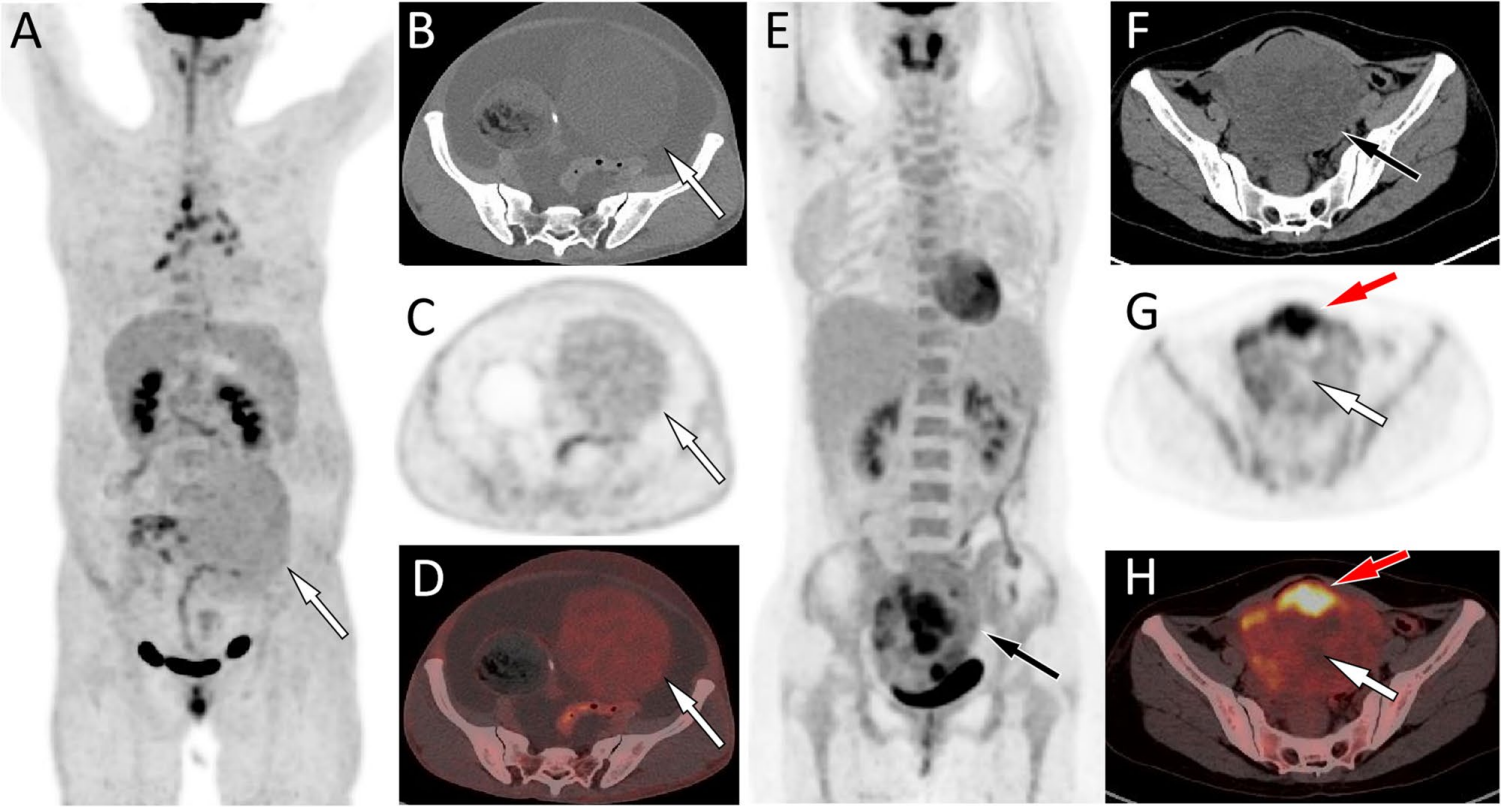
(A–D) Representative ^18^F-FDG PET/CT images of fibroma in a 59-year-old woman who admitted to hospital due to abdominal pain for 2 months. The maximum intensity projection (MIP, A) showed a slightly increased ^18^F-FDG uptake shadow in the lower abdomen (arrow). Axial CT of the corresponding site revealed a soft tissue mass of uniform density on the left side of the pelvic cavity (B, arrow). Axial PET (C) and PET/CT fusion (C) images showed a uniform and slightly increased ^18^F-FDG uptake, with a SUVmax of 2.2. (E–H) Another representative ^18^F-FDG PET/CT images of granulosa cell tumor (adults type) in a 45-year-old woman who admitted to hospital due to chest and abdominal pain for 2 weeks. The MIP (E) showed an increased ^18^F-FDG uptake shadow in the lower abdomen (arrow). Axial CT showed that the shadow was located in the pelvic cavity and was a soft tissue density mass (F, arrow). Axial PET (G) and PET/CT fusion (H, arrow) images showed varying degrees of increased uptake of ^18^F-FDG in the solid components of the tumor, with a SUVmax of 6.8 (red arrow), while the cystic area did not uptake ^18^F-FDG (white arrow). From these two representative cases, it can be seen that the SUVmax of granulosa cell tumors is significantly higher than that of fibromas.
